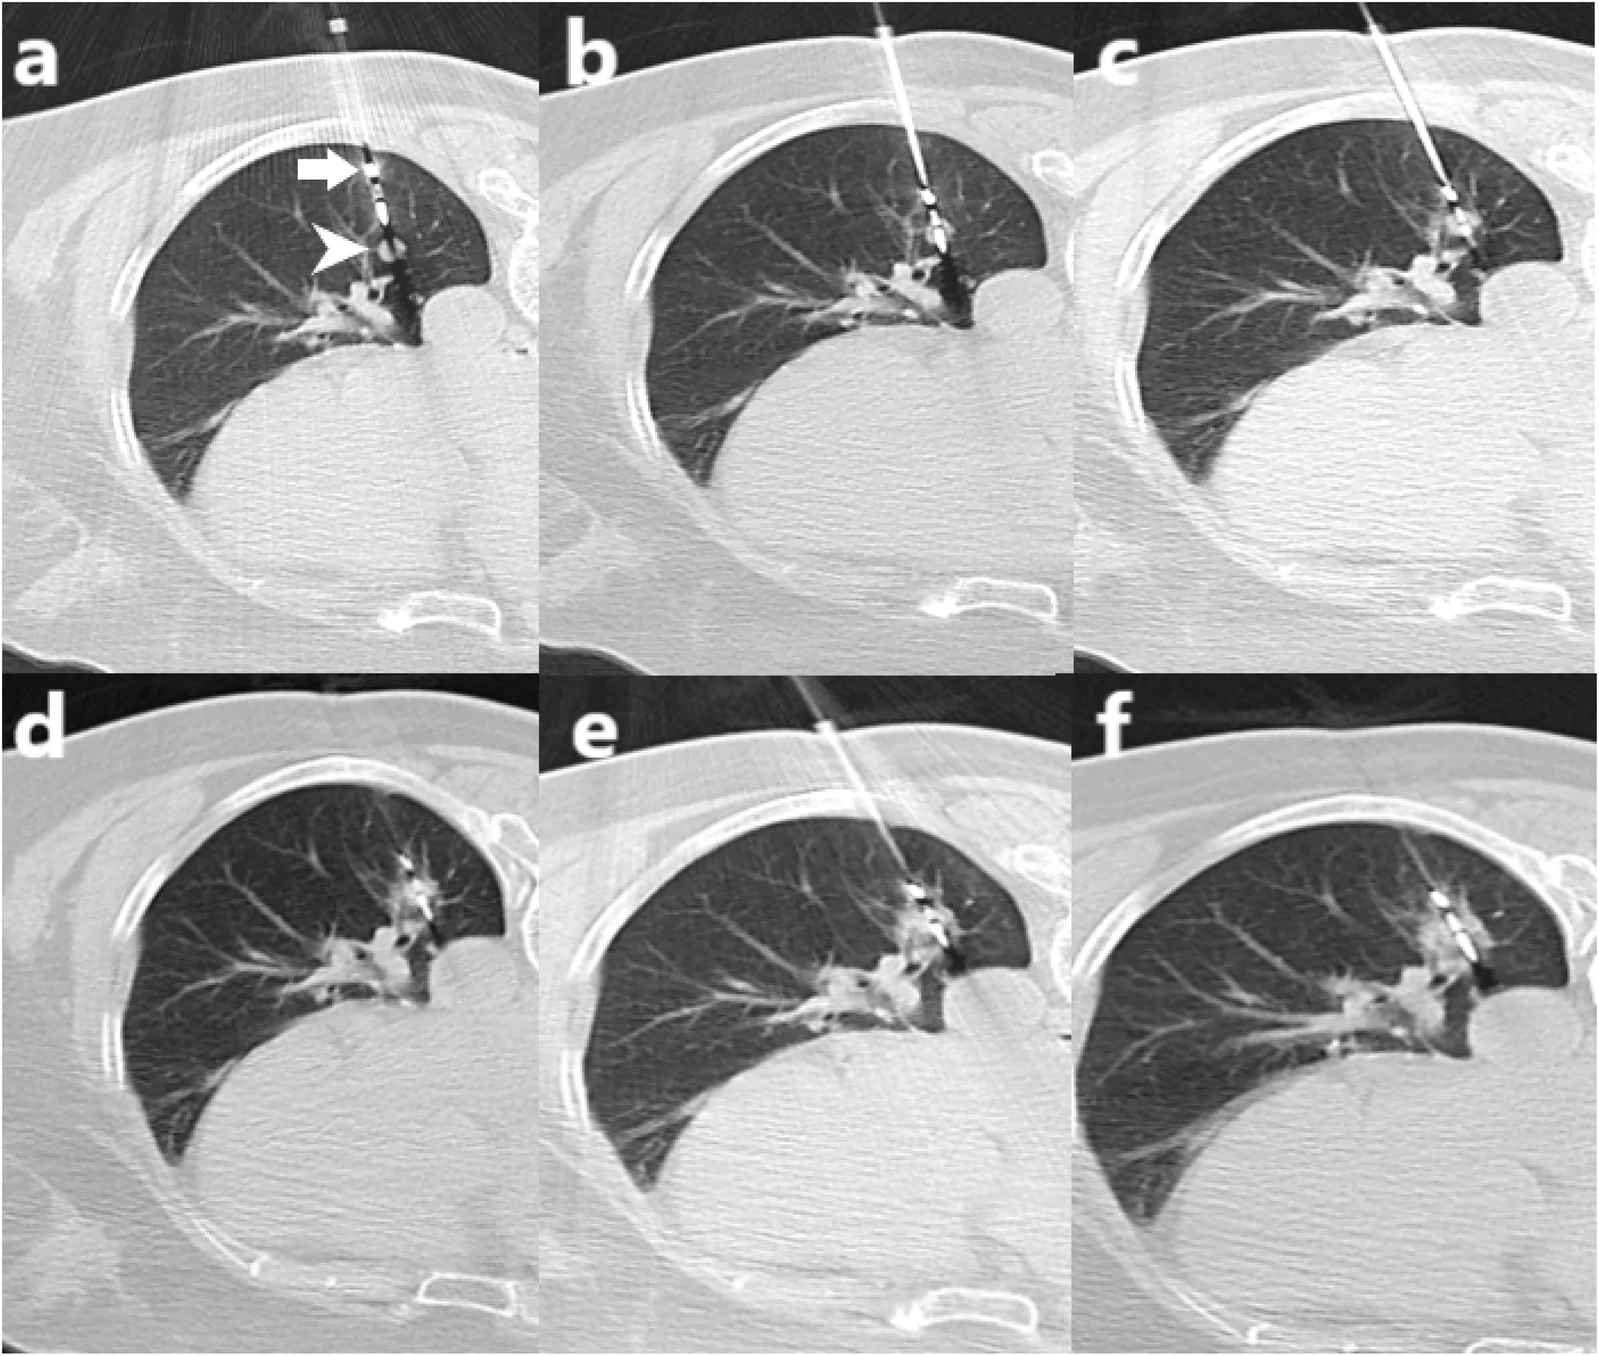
Same-level intraprocedural axial chest CT (lung window). (a,b) The needle (arrow) advances along the same track to the target (arrowhead). (c–e) Scans after each of three ablation cycles show no circumferential ablation margin in the target zone. (f) Delayed observation scan before withdrawal.
